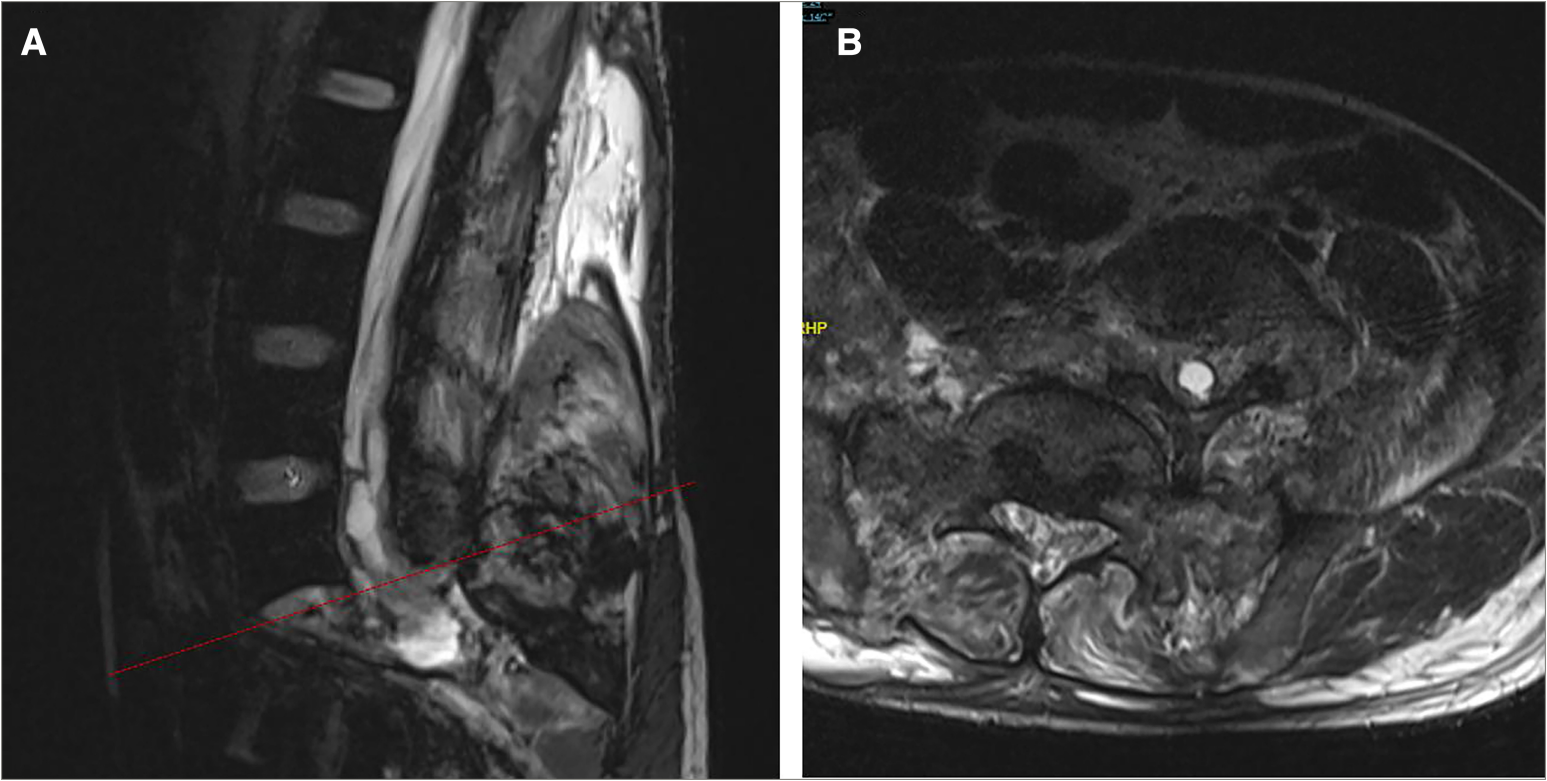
Sagittal (A) and cross-sectional (B) MRI images showed the dural tear and cerebrospinal fluid leakage. MRI, magnetic resonance imaging.
